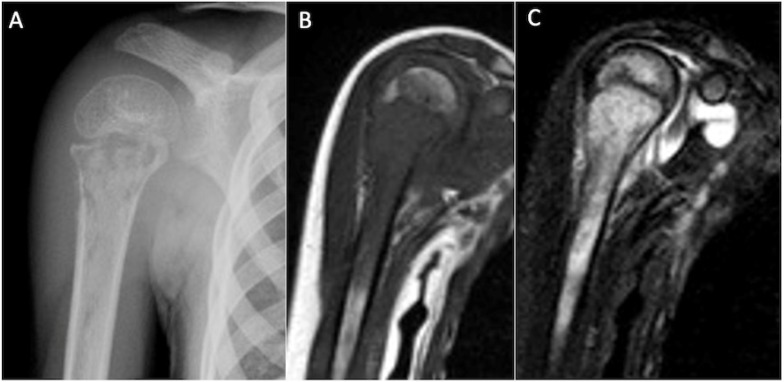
Anteroposterior radiograph (A) and coronal T1-weighted (B) and STIR (C) MRI showing Langerhans cell histiocytosis of the proximal humeral metadiaphysis, characterised by a permeative pattern of lysis and florid oedema throughout the affected medulla and adjacent soft tissues.
